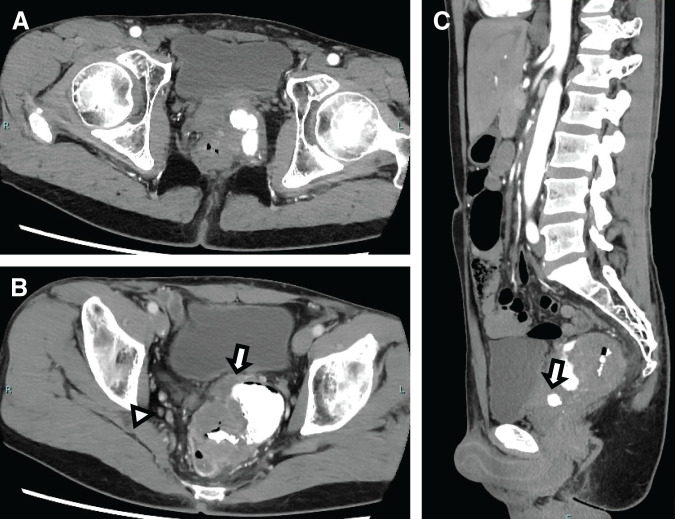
CT scan findings. (A) Horizontal image. There is a lobulation-like tumor on the left pelvic cavity with density of calcification and air. (B) Horizontal image. The tumor connects to the prostate, indicating direct invasion (arrow). Right-sided lateral lymph node shows a swelling (arrowhead). (C) Sagittal image. The mass also contacts with the urethra in the prostate, indicating fistulation (arrow).
